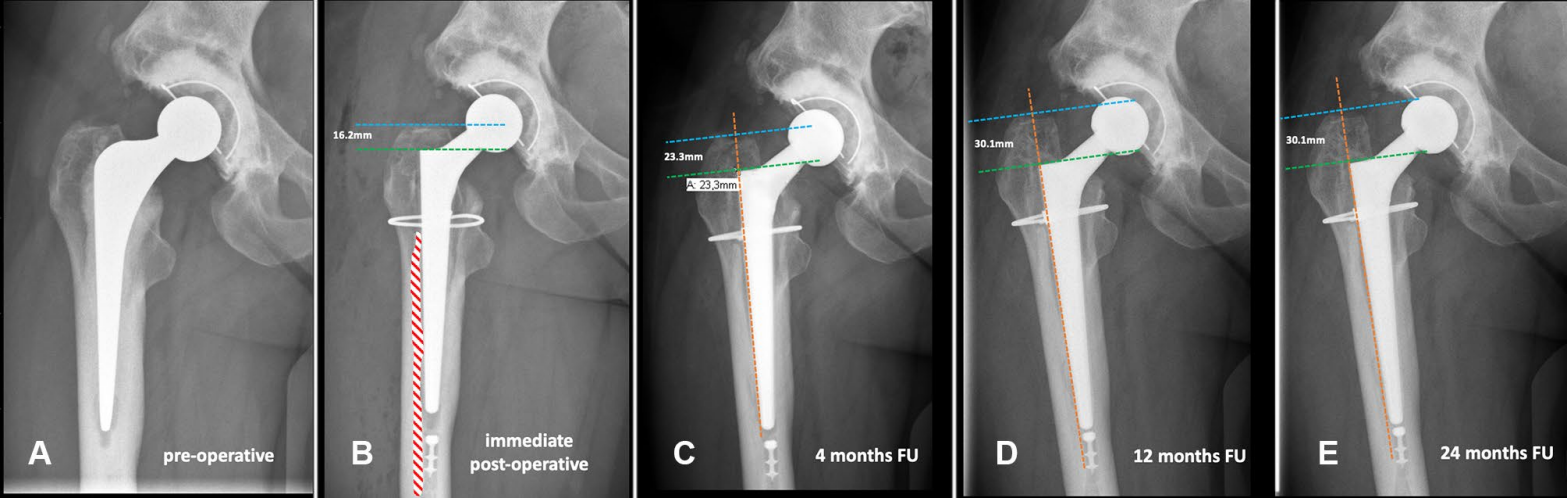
A–E show progressive subsidence of the revised stem due to insufficient cement removal (red dotted area in B). The reason for the revision was aseptic loosening. A shows a pre-operative X-ray. B shows immediate post-operative X-ray control. Subsidence was 7.3 mm at 4 months of FU (C), and 13.9 mm at 12 and 24 months of FU (D–E). *FU*  follow-up
